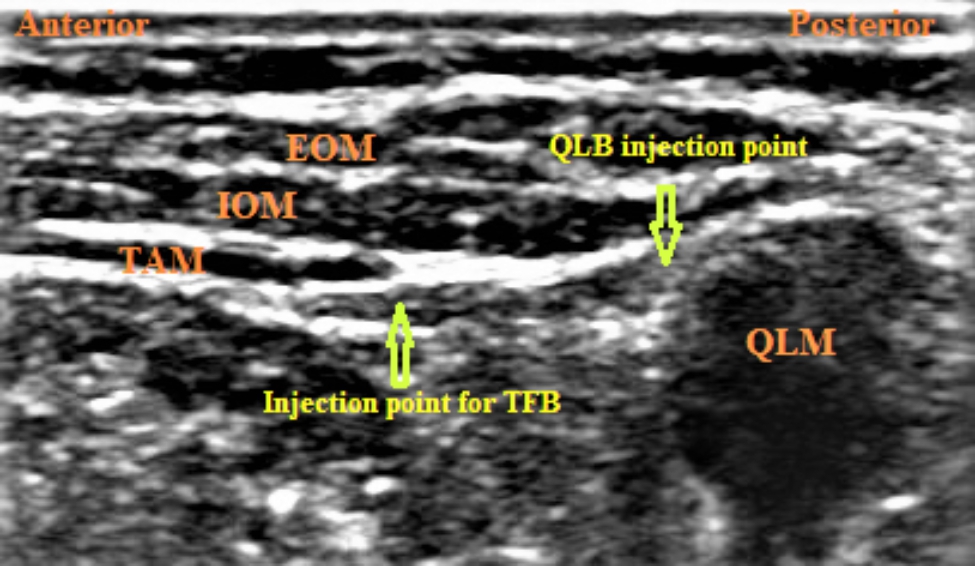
Ultrasonographic images showing the anatomy of abdominal wall muscles and the target points of injection for transversalis fascia plane block (TFB), and quadratus lumborum (QLB); EOM: external oblique muscle; IOM: internal oblique muscle; TAM: transversus abdominis muscle; QLM: quadratus lumborum muscle
